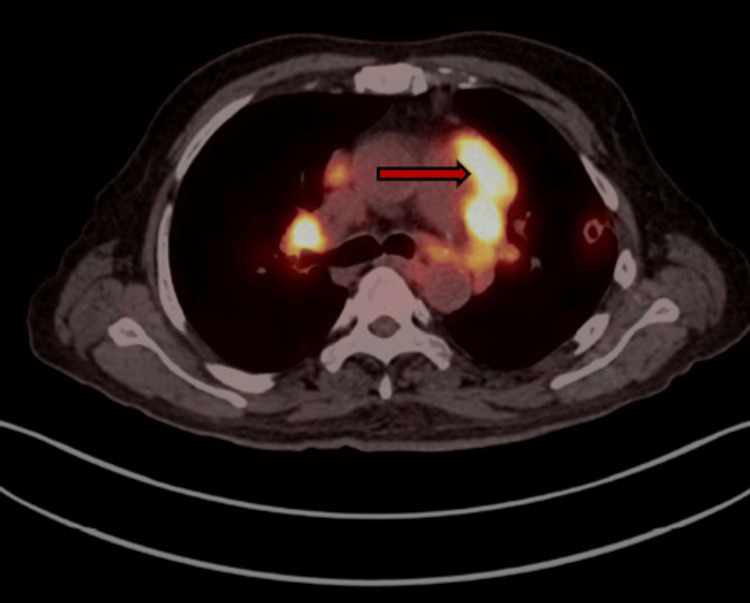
Axial fused ¹⁸F-fluorodeoxyglucose (FDG) positron emission tomography (PET)-computed tomography (CT) image at the level of the mediastinum demonstrating enlarged FDG-avid prevascular lymph nodes as shown by the red arrow
